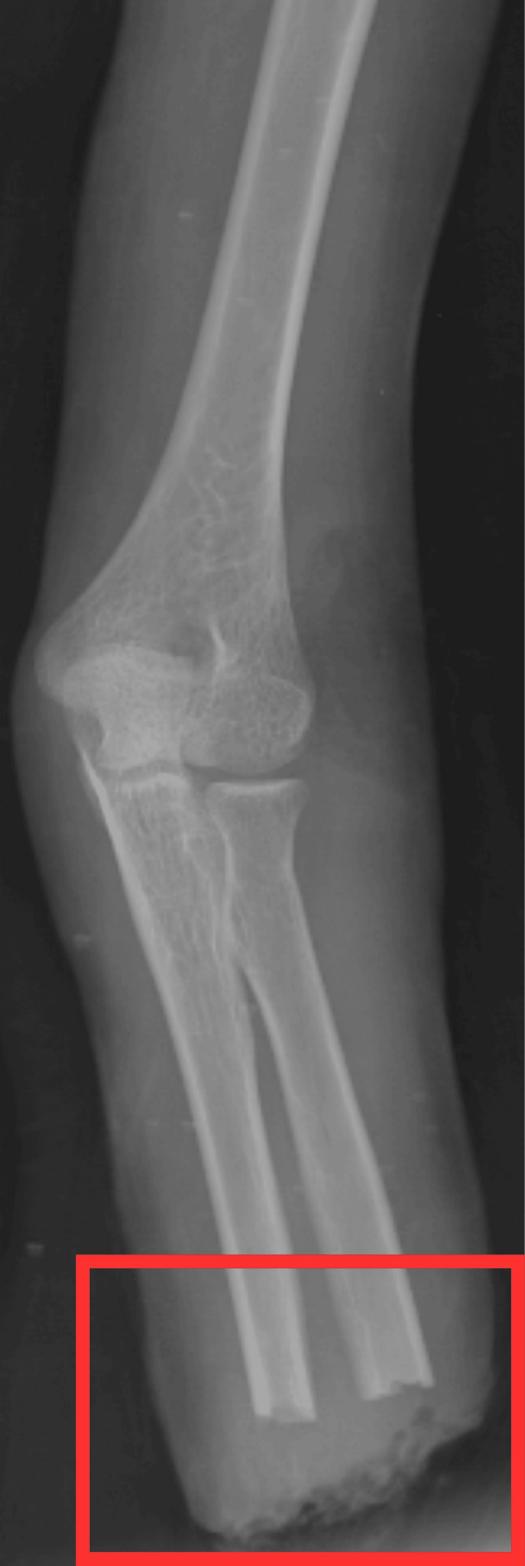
Post-operative X-ray of left amputated upper limb The red rectangle shows the transradial amputation of the right upper limb.
